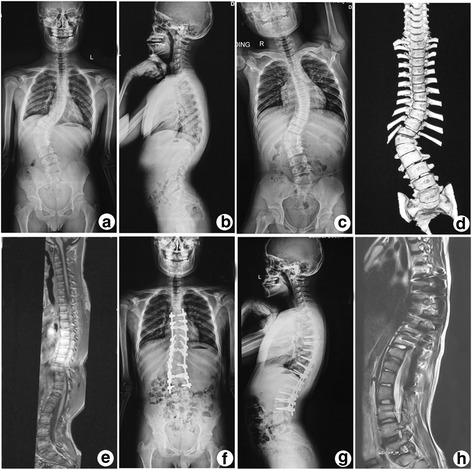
Case 7. A 15-year-old boy presented scoliosis combined with asymptomatic tethered cord. The pre-operative radiograph and CT (Fig. 2
